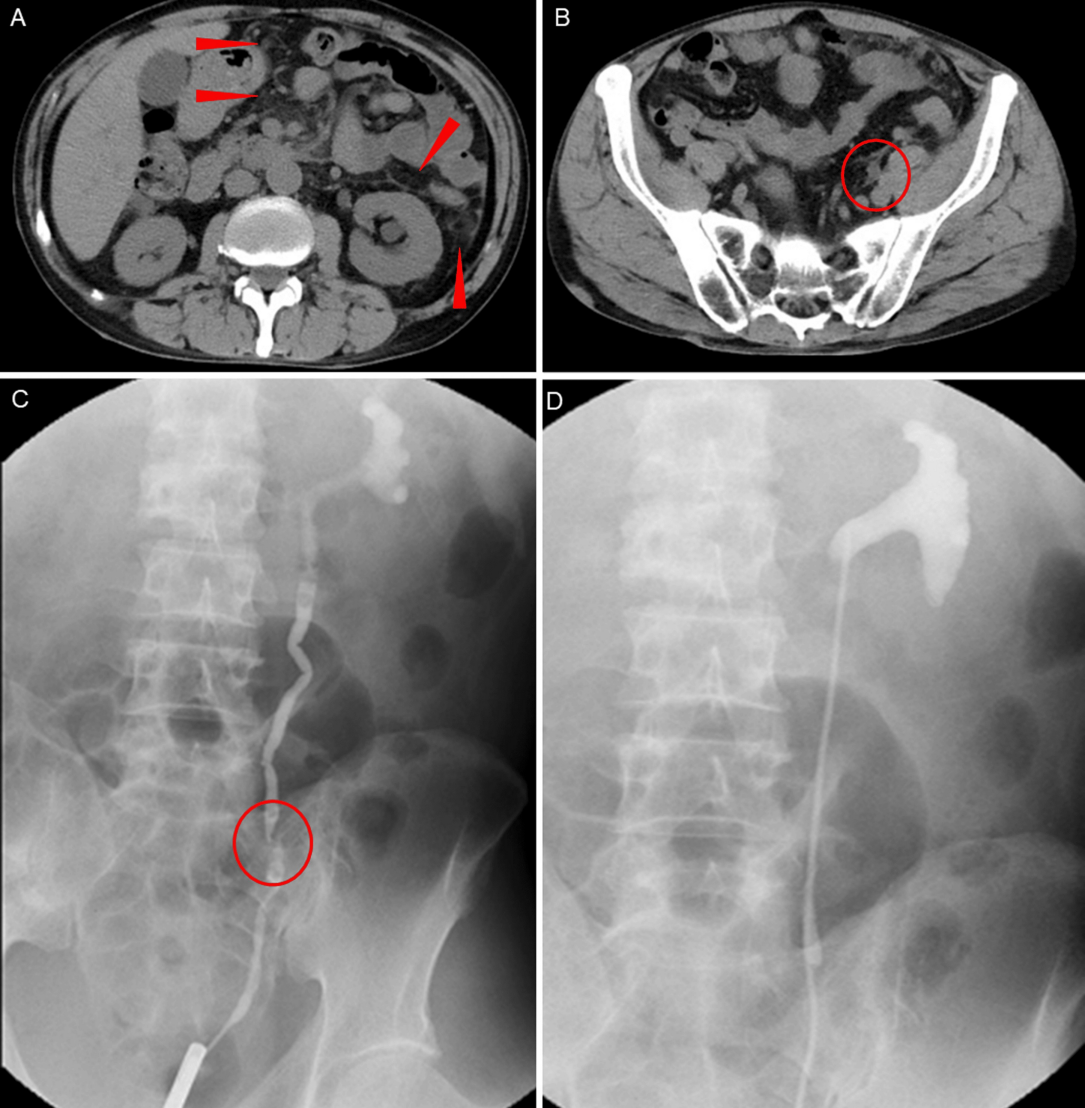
Radiological findings of left-sided malignant ureteral obstruction caused by retroperitoneal dissemination and its improvement after metallic ureteral stent placement (A) Noncontrast abdominal computed tomography showing left hydronephrosis and retroperitoneal fat stranding due to retroperitoneal dissemination (arrowheads). (B) In the pelvis, a soft-tissue density lesion is seen along the left ureter (circle), raising suspicion of impaired urinary flow caused by retroperitoneal dissemination. (C) Retrograde pyelography demonstrating a focal stricture of the mid-left ureter (circle), consistent with malignant ureteral obstruction secondary to retroperitoneal dissemination. (D) After administering a sacral block, a metallic ureteral stent (tumor stent, 7 Fr, 22 cm) was placed using a rigid cystoscope. A sensor guidewire initially failed to pass through the stricture; however, an angled hydrophilic guidewire was successfully advanced across the lesion, which subsequently allowed passage of the sensor guidewire
